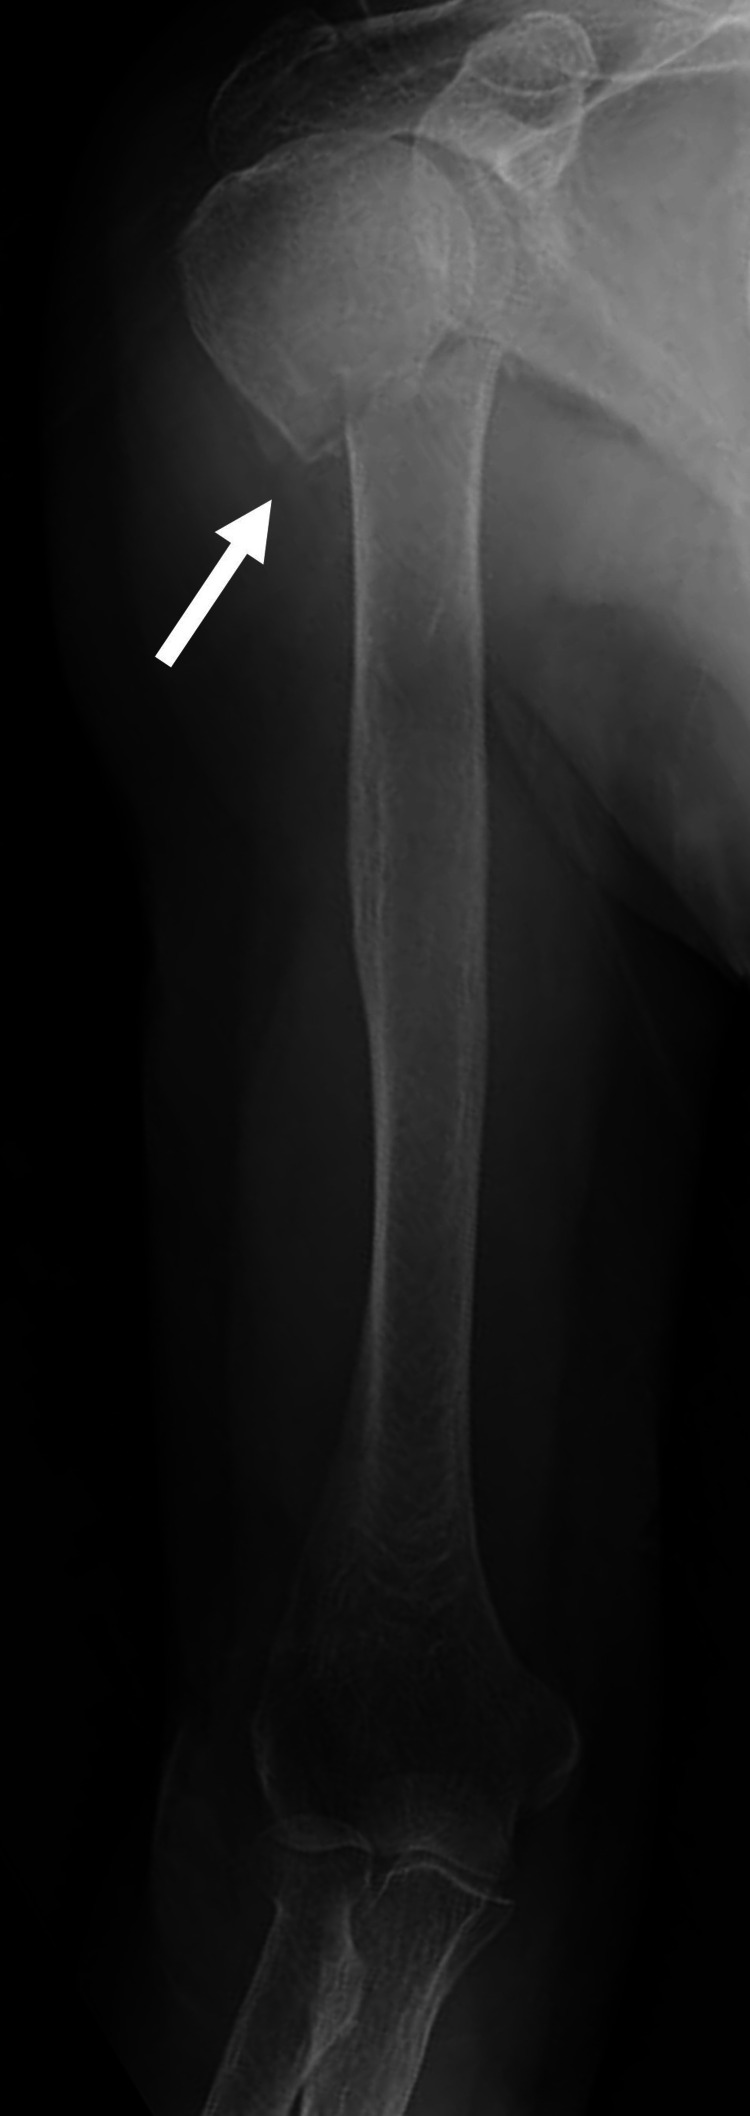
X-ray study of the humerus on arrival X-ray showed a right proximal fracture (arrow)
